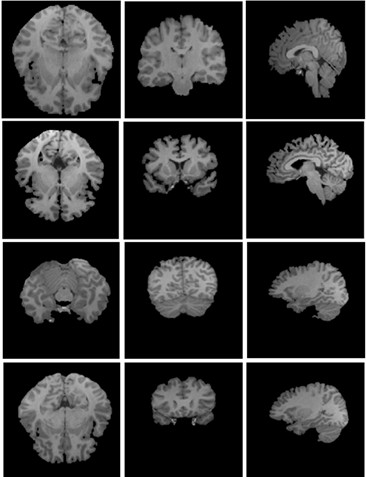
Brain extraction results of four normal T1-weighted MR brain images. Columns from left to right is shown in axial, coronal and sagittal orientations respectively.
